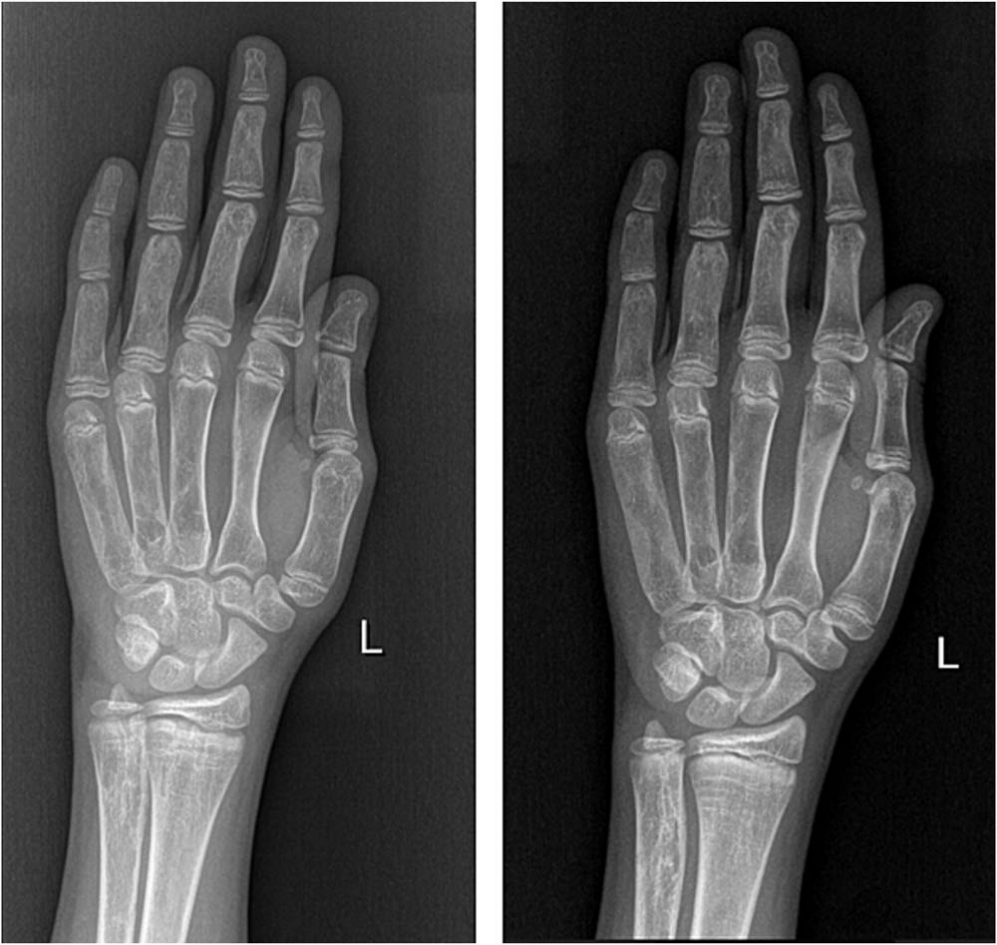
Hand X-rays 8 mo before (left) and 8 mo after starting of burosumab (right) showing mild improvement of rickets.
